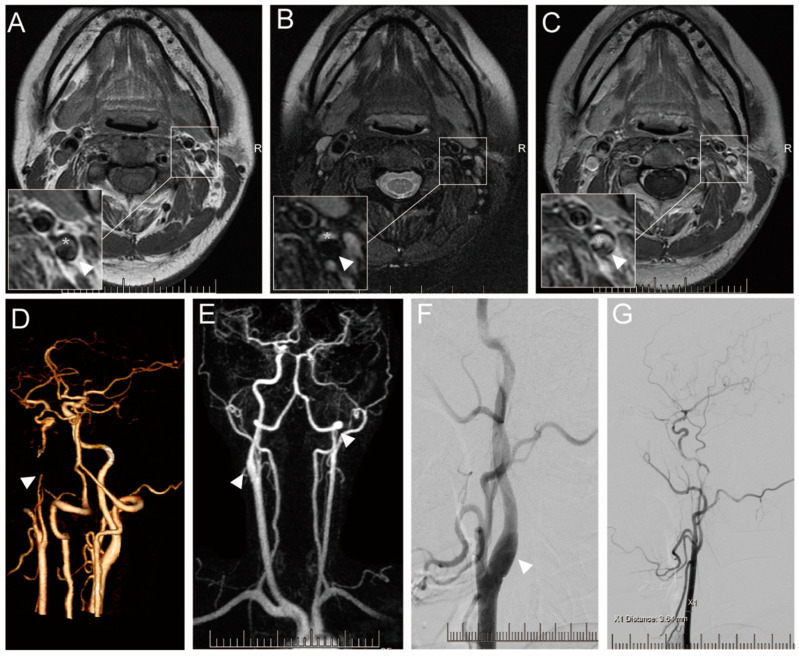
Imaging results of a 36-year-old female with SCCAD. Informed consent was obtained from the patient for the inclusion of their data in this study. Axial T1-weighted (A), T2-weighted (B), and enhanced T1-weighted (C) showing left ICA with hematoma within vascular wall (white asterisk), a true stenotic lumen (white arrowhead). CTA (D) shows the occlusion of the left ICA (white arrowhead). HR-MRA (E) showing the occlusion of left ICA (white arrowhead) and limited enlarged right ICA with hematoma within the vascular wall. DSA offers the local vascular dissection in the right ICA (F) and recanalization of the left ICA (G).
